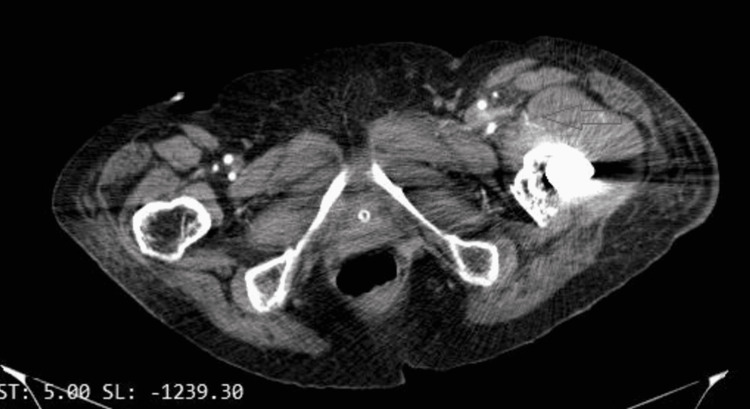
Axial computed tomography angiography image demonstrates the small extravasation (red arrow).
